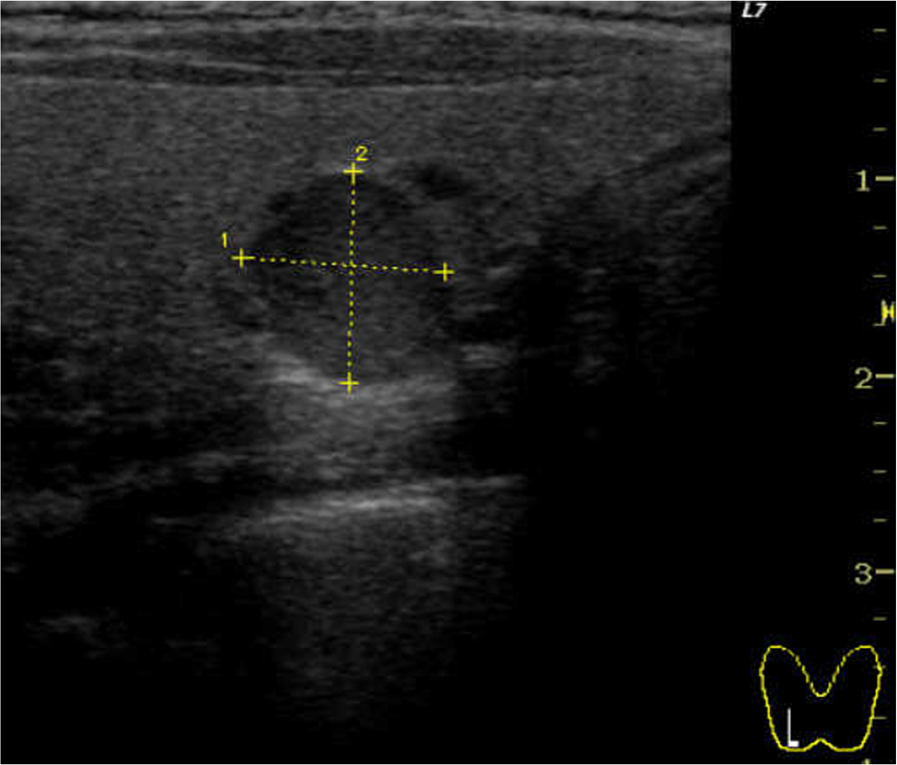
Cervical ultrasonography. A mass shadow with a 1-cm diameter at a site corresponding to the right inferior pole of the thyroid gland.
